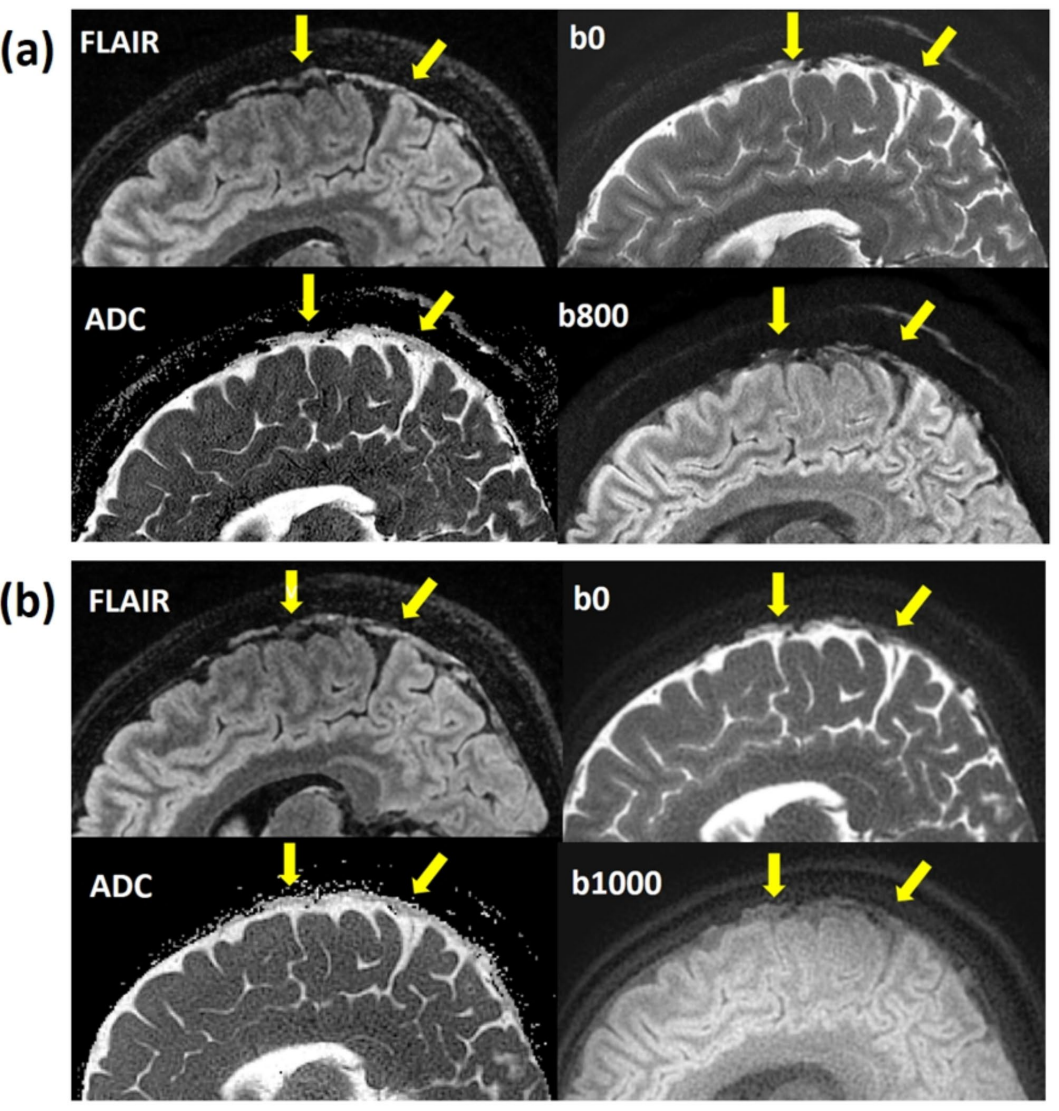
(a) The sagittal view displayed the PSD on T2 FLAIR image (up left), MUSE DWI b0 (up right), MUSE DWI b800 (down right), and MUSE ADC map (down left) as indicated by arrows. (b) The sagittal view displayed the PSD on T2 FLAIR image (up left), PROPELLER DWI b0 (up right), PROPELLER DWI b1000 (down right), and PROPELLER ADC map (down left) as indicated by arrows. The ADC of the PSD appeared darker than that of the CSF.
